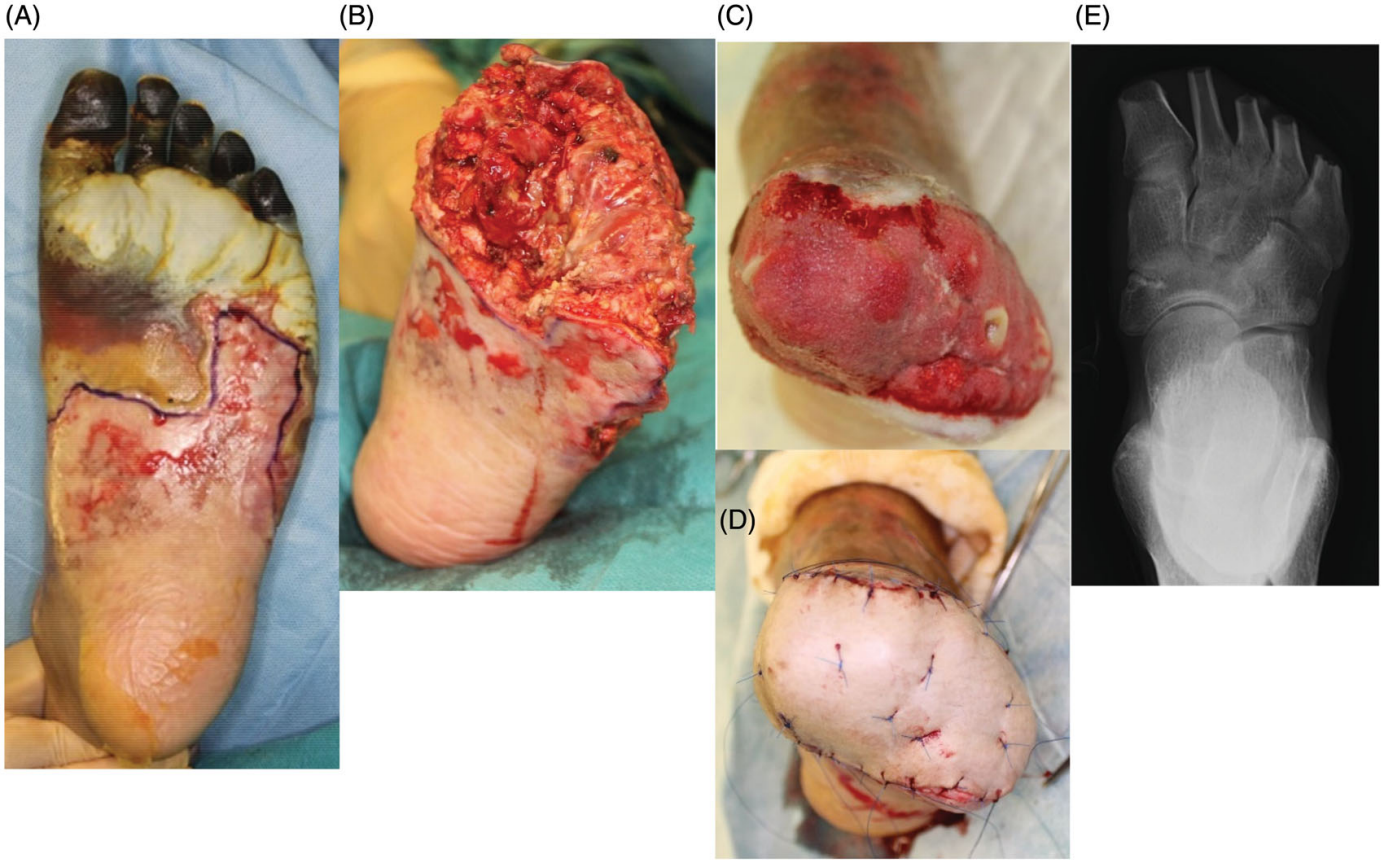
The left foot was amputated at the level of the metatarsal bone. All necrotic tissue was excised. Negative pressure wound therapy (NPWT) was applied to any wounds associated with exposed bone to speed up the healing process and cover the bone with granulation tissue as a wound bed preparation. After three weeks, there was good granulation, with the bone almost buried. Finally, a partial-thickness skin graft was applied to the wounds. (A) Before amputation, (B) after amputation, (C) condition at three weeks after NPWT, (D) skin graft on wound, (E) X-ray findings after surgery.
